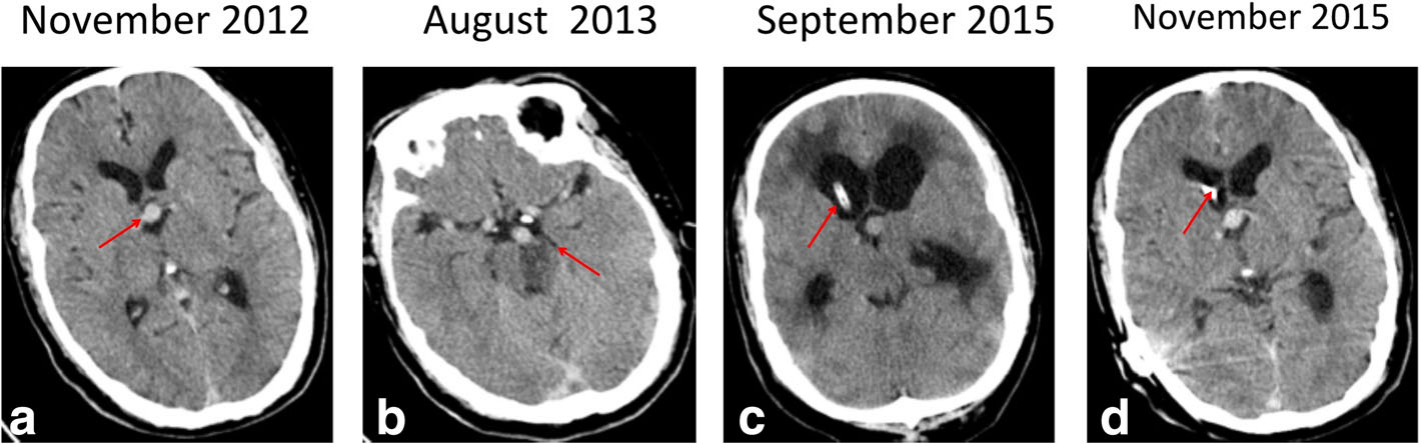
Serial axial non-enhanced CT scans. (a) Upward displacement of the third ventricle (red arrow) by a markedly ectatic BA. (b) Left pontine infarction compressed by the ectatic BA (red arrow). (c) Bilateral dilated ventricles and low density lesions anterior and around the lower horns on the second day after ventriculoperitoneal shunt placement (red arrow). (d) Reduced ventricular dilation and disappearance of low density lesions around the ventricle horn (red arrow) one month after shunt placement
